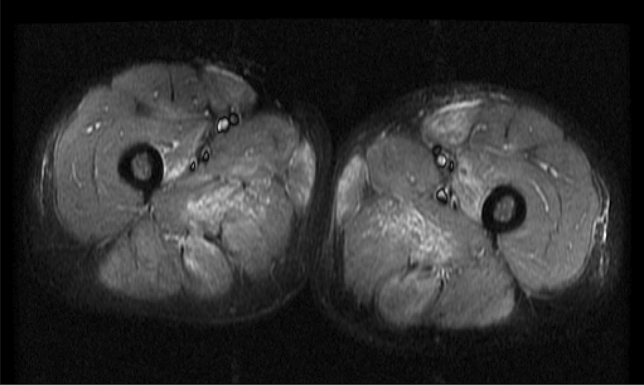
Case 1. Fat-suppressed T2 MRI thighs, showing increased signal in adductor magnus, gracilis, vastus intermedius and rectus femoris muscles
